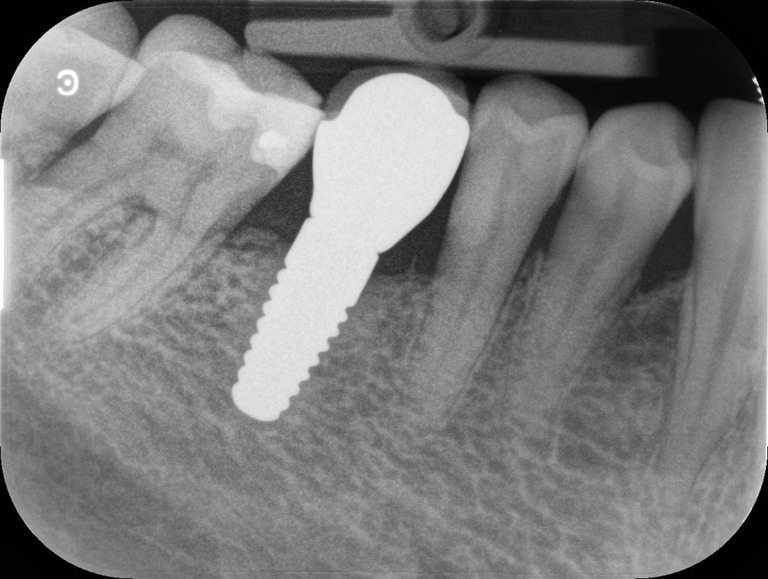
Peri-apical radiograph of a zirconia implant-supported single crown
